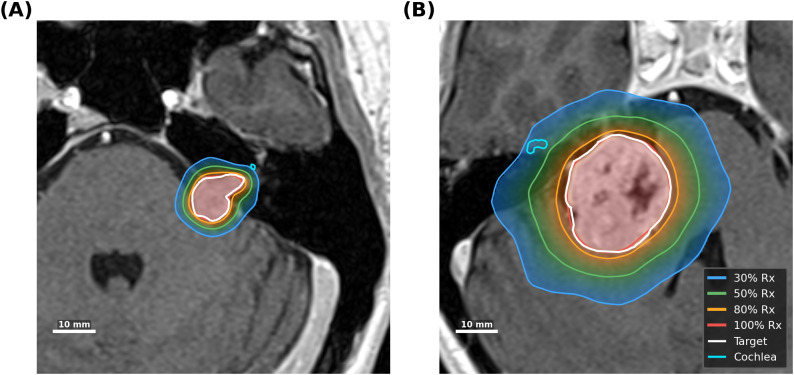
Representative axial dose distributions from VS-SEG clinical cases. (A) VS-SEG-023 (TV = 0.55 cc, dDGI = 0.238 mm): a small vestibular schwannoma with tightly spaced isodose lines indicating a steep dose gradient. (B) VS-SEG-234 (TV = 7.18 cc, dDGI = 0.562 mm): a large target with wider isodose spacing reflecting a shallower gradient. Solid lines indicate isodose levels (30%, 50%, 80%, and 100% of Rx), the target volume (white), and cochlea (cyan). T1-weighted MR images are shown as background. The scale bar represents 10 mm.
